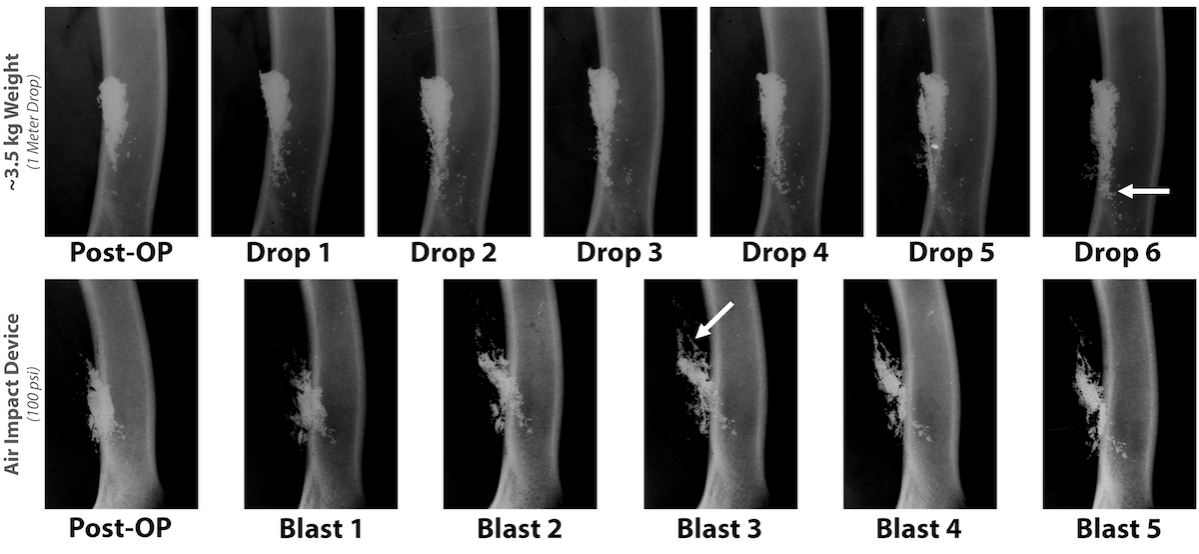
Radiograph demonstrating that following air impact device exposure or blunt force trauma, femora were intact and not fractured. In addition, images show dispersion of Cobalt-Chromium beads (white).
